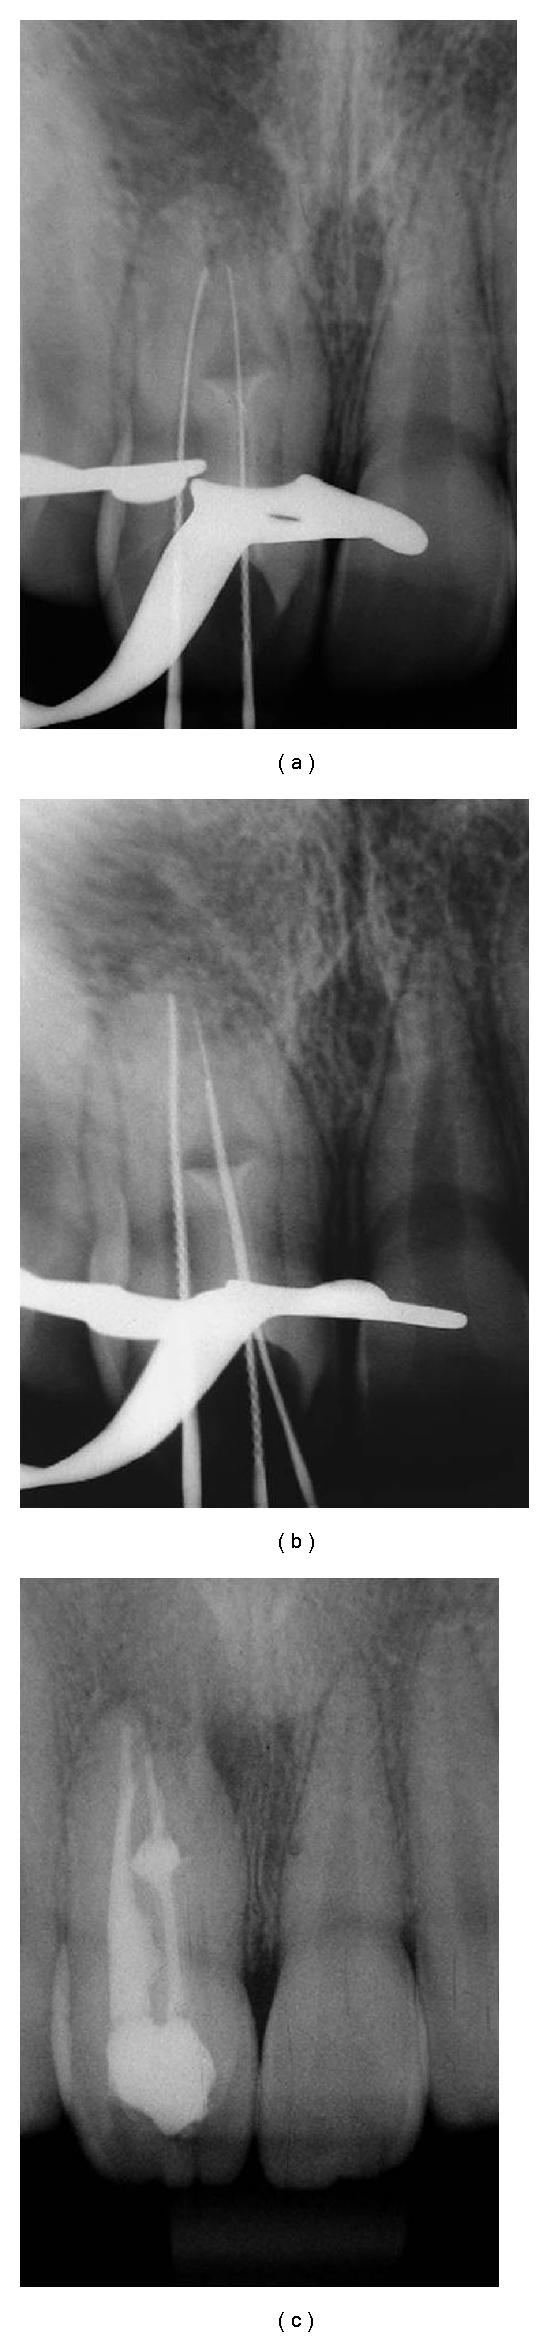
Endodontic treatment. (a) and (b) The work lengths of the two canals were established at one millimeter from the radiographic apex. (c) Root canal filled.
